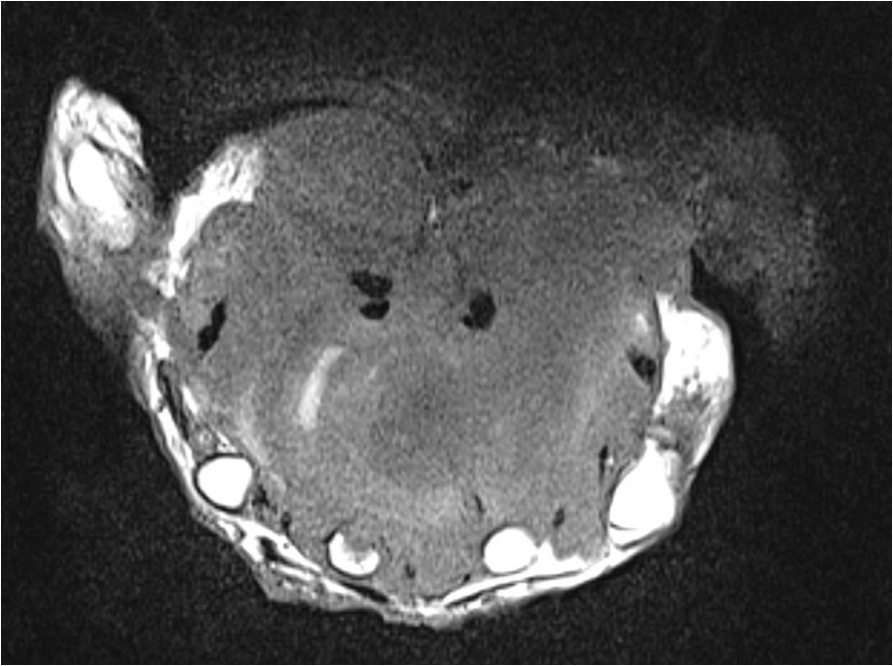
Magnetic resonance image of a transverse section of the hand showing a large mass around the palmar structures of the hand, but with no bone involvement.
